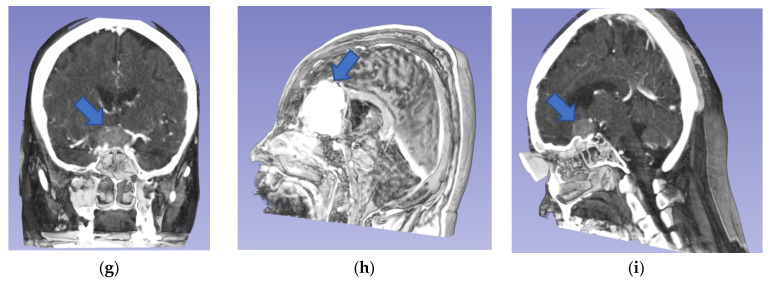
Preoperative 2D and screen 3D images (from CT and MRI modalities) of patients with anterior skull base meningiomas (blue arrows). (a) Axial 2D-CTA image presenting planum sphenoidale meningioma. (b) Sagittal 2D-CTA image presenting olfactory groove meningioma. (c) Coronal 2D-CTA image presenting tuberculum sellae meningioma. (d) Axial 2D-MRI image presenting olfactory groove meningioma. (e) Sagittal 2D-MRI image presenting anterior clinoidal meningioma. (f) Coronal 2D-MRI image presenting frontobasal meningioma. (g) Coronal screen 3D-CT image presenting tuberculum sellae meningioma. (h) Sagittal screen 3D-MRI image presenting olfactory groove meningioma. (i) Sagittal screen 3D-CT image presenting anterior clinoidal meningioma. 2D, two-dimensional; 3D, three-dimensional; CT, computed tomography; CTA, computed tomography angiography; MRI, magnetic resonance imaging.
